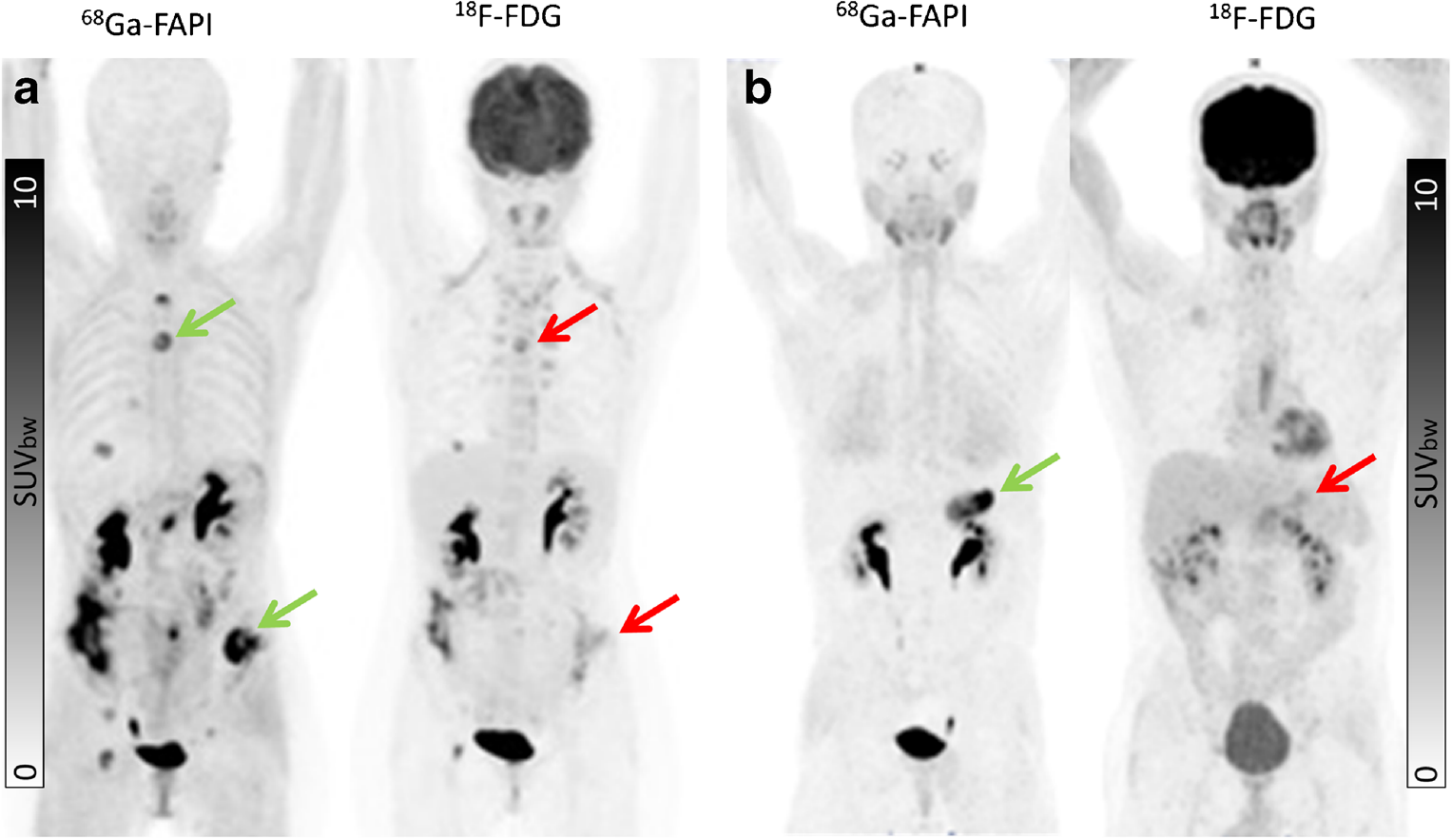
Intra-individual comparison of ^18^F-FDG and ^68^Ga-FAPI in two patients with ovarian cancer (a) and pancreas cancer (b), respectively. Both present with strong ^68^Ga-FAP uptake in the primary and metastatic lesions while only slight to moderate uptake on ^18^F-FDG PET/CT (arrow: green (^68^Ga-FAPI) and red (^18^F-FDG)
